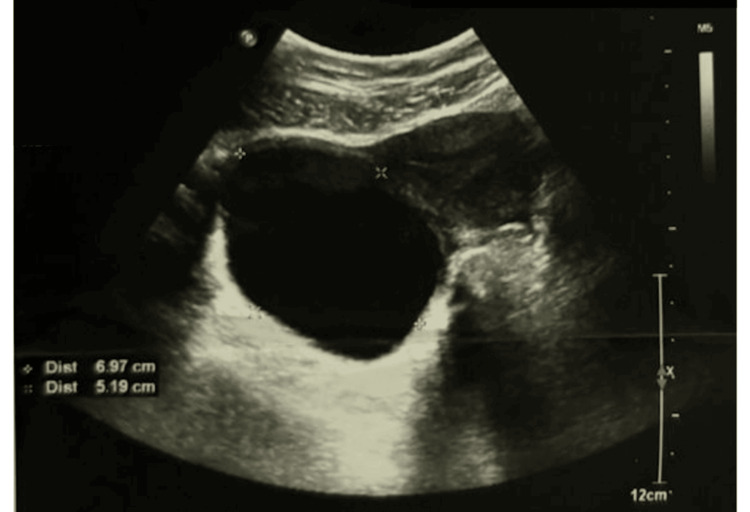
Pelvic ultrasound The right ovary with hyperechoic inclusions with clearly contoured anechoic formations, measuring 70 x 62 x 79 mm. Normal follicles are identified. No free pelvic fluid is seen in the cul-de-sac.
